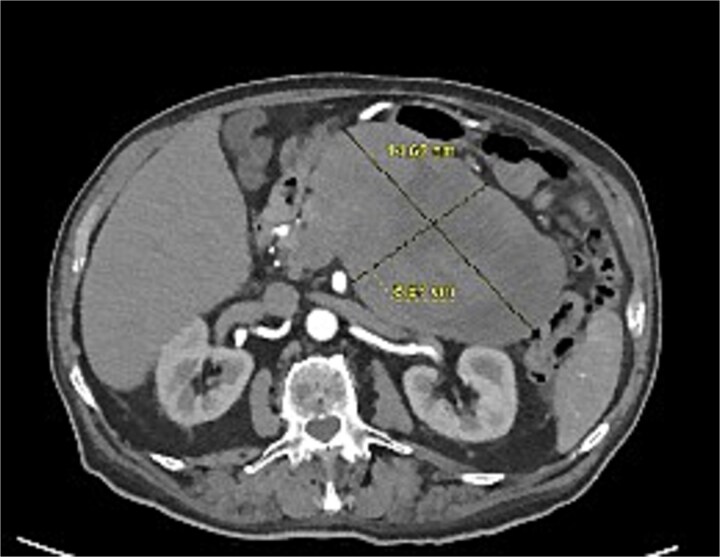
Transverse high resolution CT of the PACC prior to surgery.
